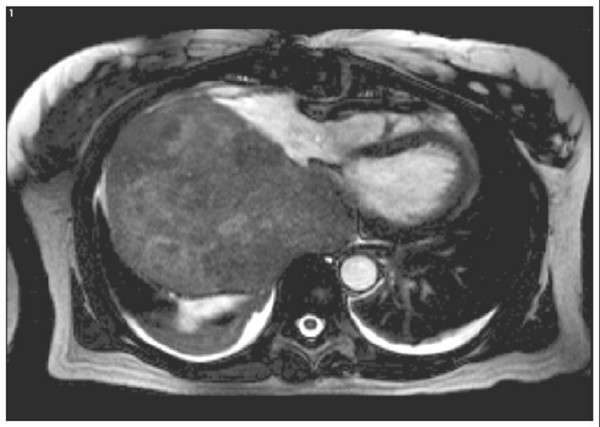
Magnetic resonance image demonstrating huge right sided mass within the chest.
